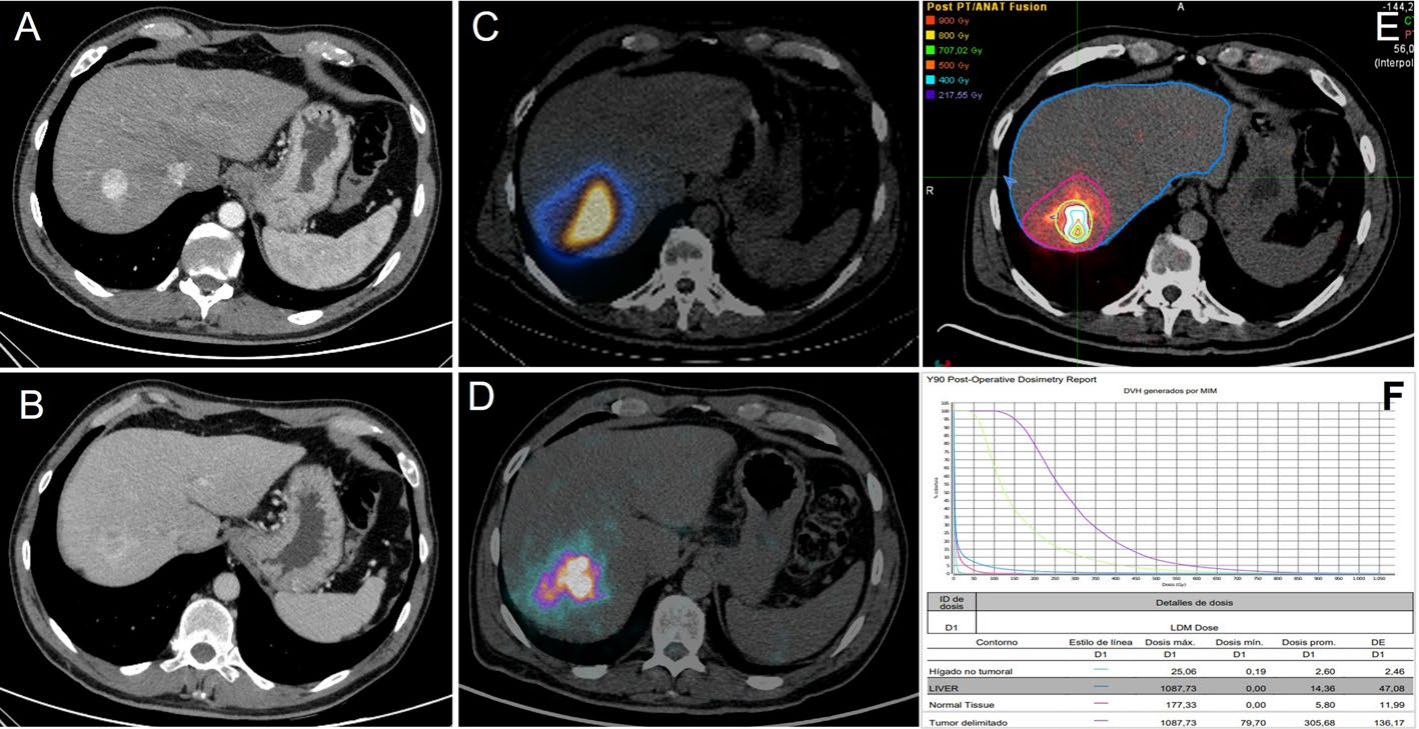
Diagnostic CT in arterial (A) and portal (B) phases, showing a 23-mm hypervascular nodule in segment VII with contrast washout, diagnosis of HCC. Tc-99 m MAA SPECT/CT (C) and Y-90-PET/CT (D) image after administration of 0.78 GBq of glass microspheres, showing selective deposition of radioparticles on the tumor and, to a lesser extent, on the rest of the segment. Dosimetric study (E and F) using MIM software, maximum tumor dose 1087 Gy, mean dose 305 Gy
